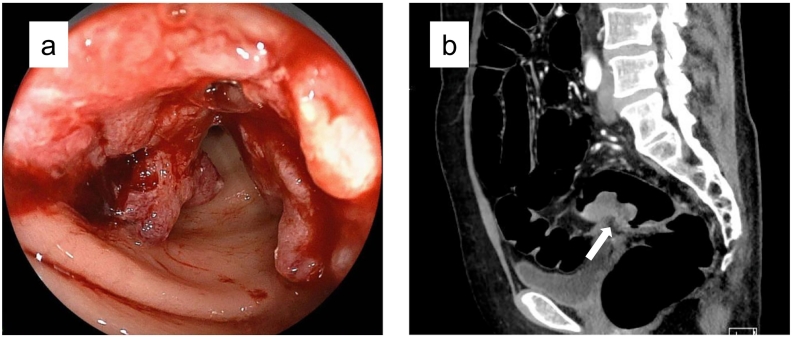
Rectosigmoid colon cancer (arrow) was diagnosed by colonoscopy (a) and computed tomography (b) scan sixteen months before presentation and treated with laparoscopic high anterior resection.
